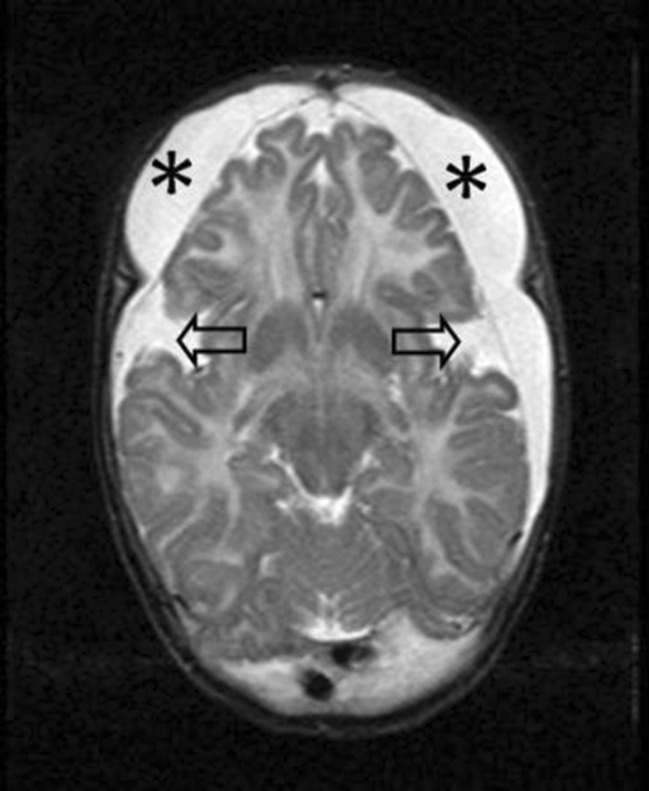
T2-weighted MRI of a boy at 6 months old (patient no. 14) with subdural collections. MRI settings: T2 SE, slice thickness 6 mm, TR: 3465, TE 150, flip angle 90°, NSA: 1. Clinical history revealed a consistent macrocephaly and mild retardation, no signs of acute neurological deterioration. MRI shows symmetrical fronto-parieto-occipital subdural collections (*asterisk*), atrophy of the frontal lobes, and bilateral wide Sylvian fissures (*arrows*). The imaging findings are consistent with GA1. The work-up for child abuse revealed no concern
